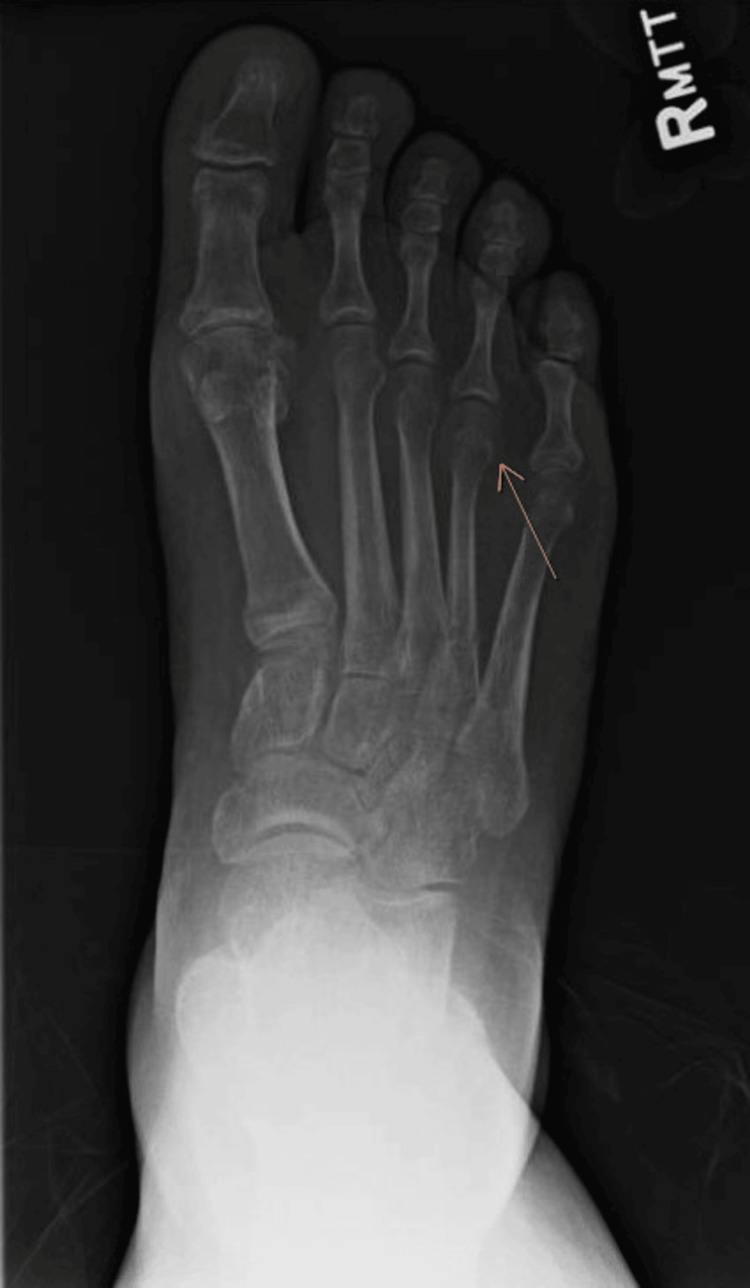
Radiograph of the right foot Demonstrates healing proximal diaphyseal fracture at the fourth metatarsal and presence of inferior calcaneal spurring with generalized osteopenia.
